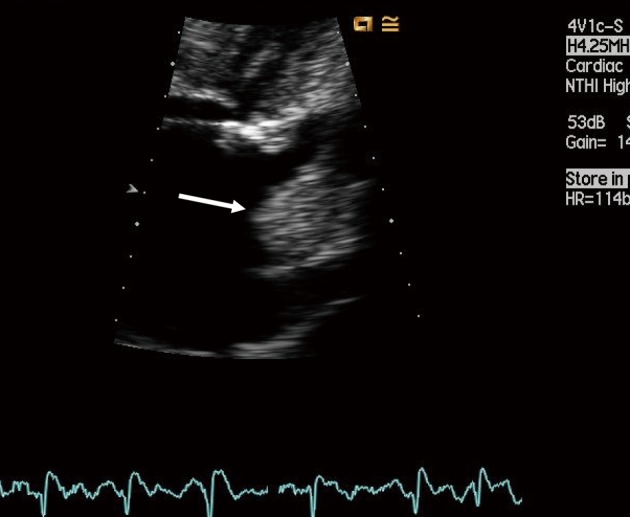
Large thrombus protruding from the left atrial appendage (arrow) as seen by transesophageal echocardiography.
